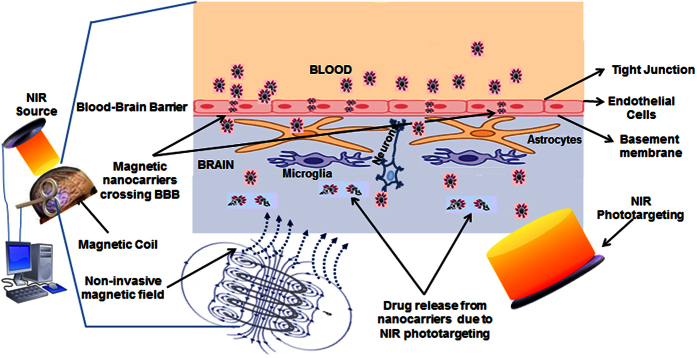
Proposed mechanism for efficacious targeting, delivery, release, and monitoring of therapeutics to brain using transient NIR photonics with MNPs for noninvasive brain targeting: An *in silico*-controlled, non-invasive magnetic force will drive MNPs across BBB and simultaneously NIR phototargeting can cause release of associated drugs (transitory optoelectronic excitation-mediated molecular vibrations on MNPs surfaces, even for short NIR laser exposure, change the charge distribution of MNPs surfaces and subsequently the ionic interaction of drugs can be broken with subsequent drug release). Also, while MNPs alone are used for MRI imaging; a “MNP-NIR sensitive fluorescent materials::core-shell” template can be an excellent use for multi-color imaging modalities. Combining fluorescent liposomes, hydrogels, carbon dots, or matrix of upconversion materials such as Erbium, Thulium, etc., can be used for this purpose because these materials can emit different color fluorescent lights depending on NIR wavelength.
